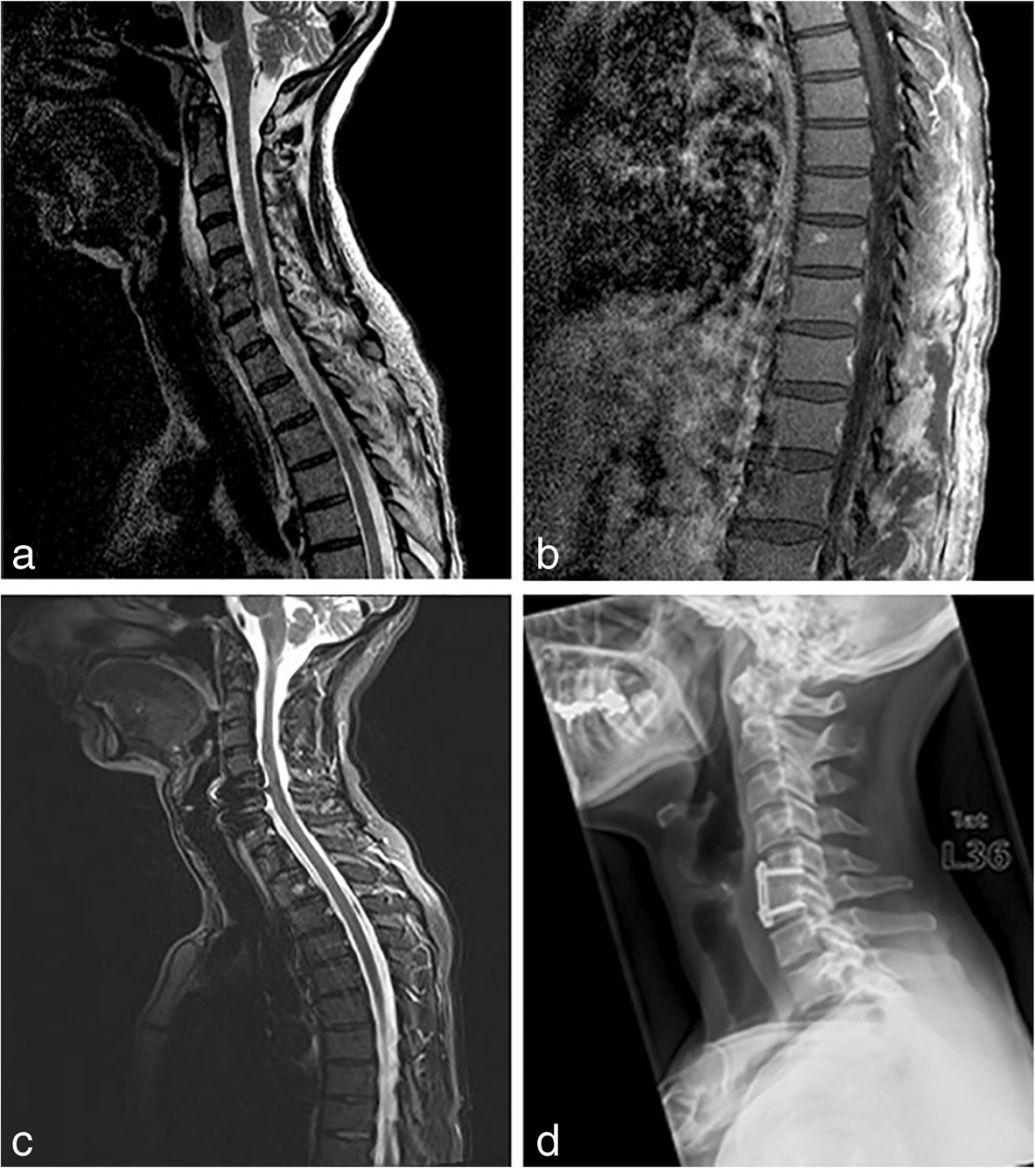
MRI of one patient showing a spondylodiscitis C5/6 with an epidural abscess (a) and thoracolumbal abscesses of paravertebral muscles (b). Follow up MRI with no signs of an ongoing inflammatory process (c) and control x-ray showing the consolidated fusion after ventral spondylodesis (d).
